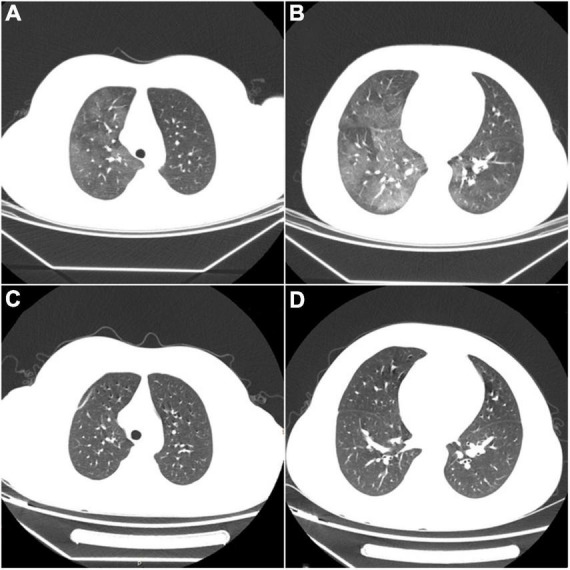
(A,B) Diffuse ground-glass opacities consistent with hemorrhagic changes in both lungs. (C,D) After treatment, repeated CT shows complete disappearance of the abnormal lesions.
